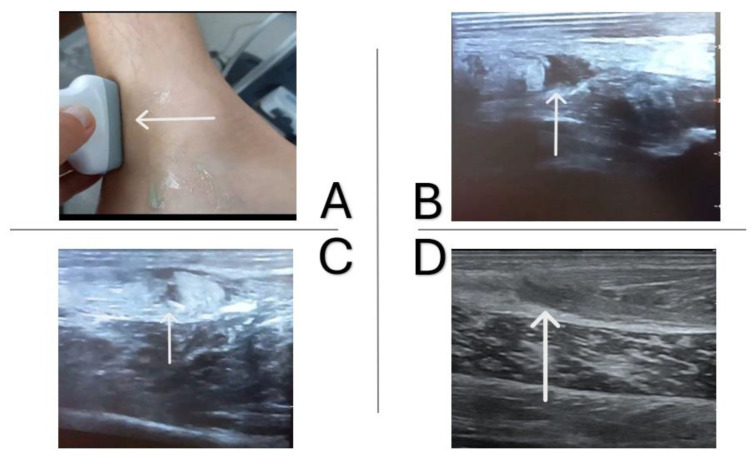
Ultrasound examination of the soleus muscle (A,B) immediately after the injury, (C) after 8 weeks of treatment, and (D) after 16 weeks of treatment.
